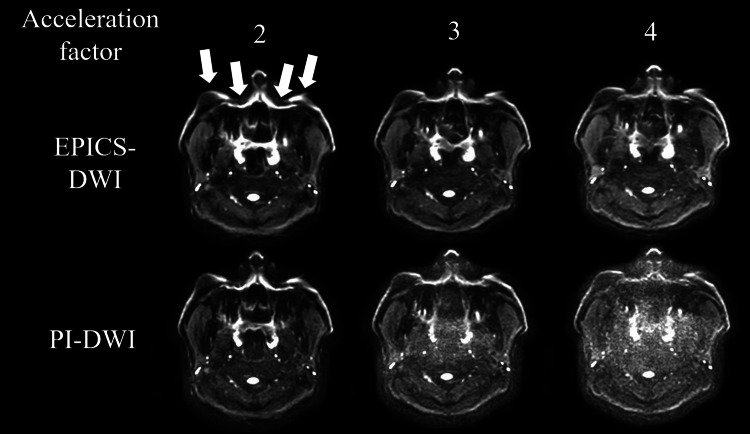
Representative case of both EPICS-DWI and PI-DWI Axial EPICS-DWIs and PI-DWIs in a 33-year-old male were presented. Particularly, EPICS-DWI with an acceleration factor of 3 demonstrated both less image noise and less image distortion. Compared to this image, PI-DWI and EPICS-DWI with an acceleration factor of 2 maintained a certain degree of image distortion (arrows). In addition, moderate or severe image noise was observed in PI-DWI with an acceleration factor of 3 and 4 and EPICS-DWI with an acceleration factor of 4
